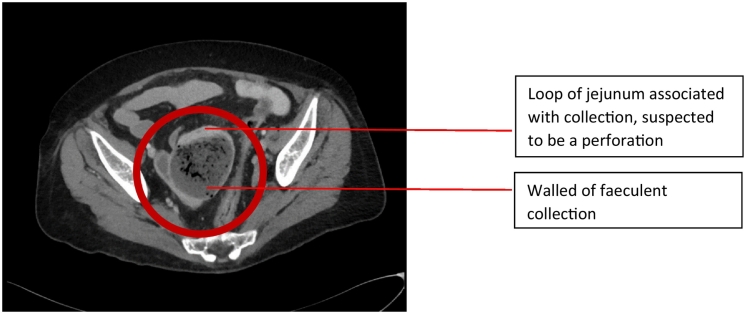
Axial view of CT scan at presentation.
